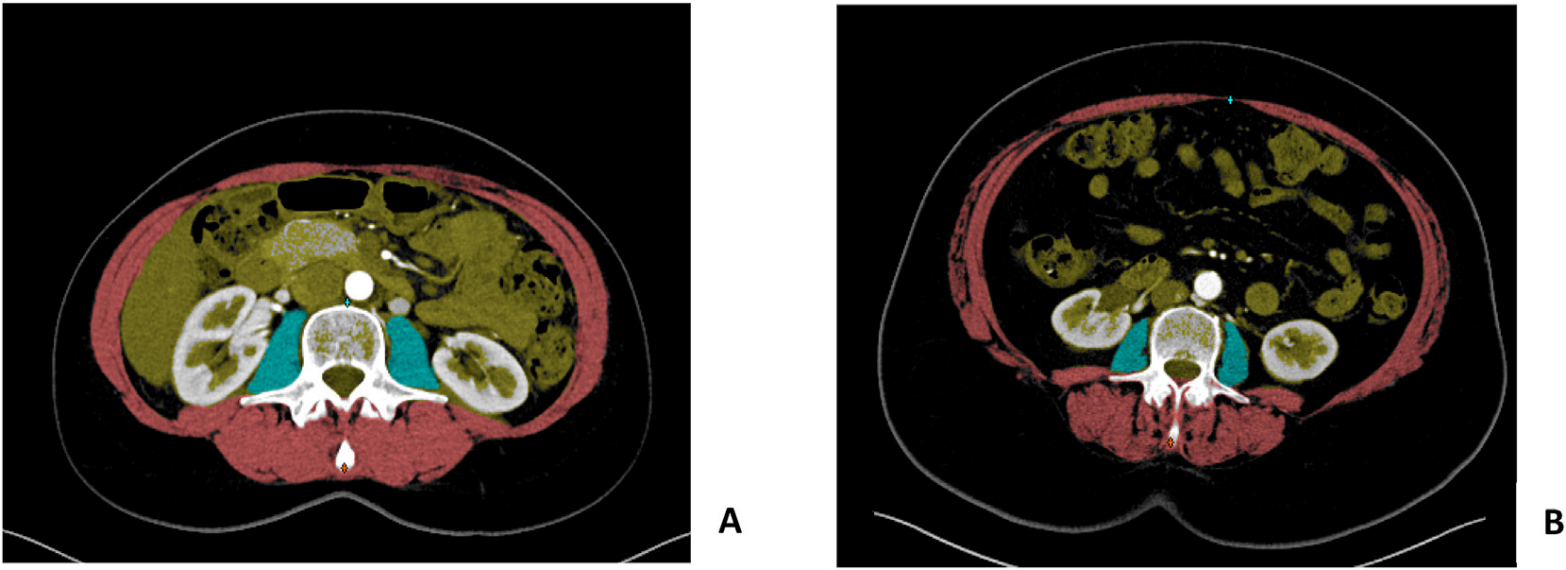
Anatomical outlines acquired with SarcoMeas of the preoperative thoracoabdominal CT-image at lumbar level L3, transverse section. These images show the method of measuring skeletal muscle density (SMD) and SMI and are an example of the anatomical variation related to muscle and fat ratio's between two women undergoing breast reconstruction. The left images (A) show an SMD above the mean (48HU), the image on the right (B) shows a low SMD (26HU). Blue areas: left and right psoas muscle. Red areas: abdominal wall, skeletal muscles. Yellow areas: intra-abdominal organs and fat.
